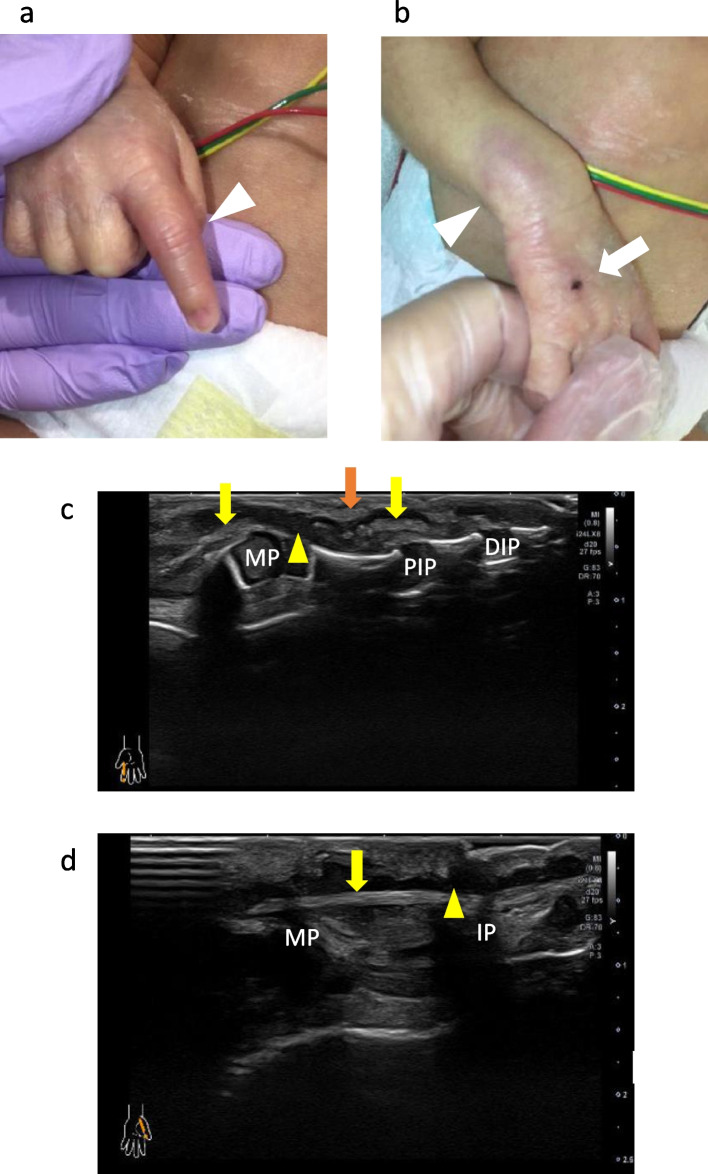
a Redness and swelling of the right index finger’s proximal interphalangeal (PIP) joint are observed (arrowhead). The PIP joint presents impairment in spontaneous flexion. b Scab formation and redness are observed around the indwelling needle removal mark on the dorsum of the right hand (arrow), and purpura is seen on the wrist (arrowhead). c Ultrasound imaging of the right index finger on the day of hospitalization. The flexor digitorum profundus tendon (yellow arrows) is torn and contracted, and the flexor digitorum superficialis tendon (orange arrow) is torn and relaxed. Fluid accumulation is seen in the tendon sheath (yellow arrowhead). d The flexor pollicis longus tendon is intact (yellow arrow), but fluid accumulation is seen in the tendon sheath (yellow arrowhead)
